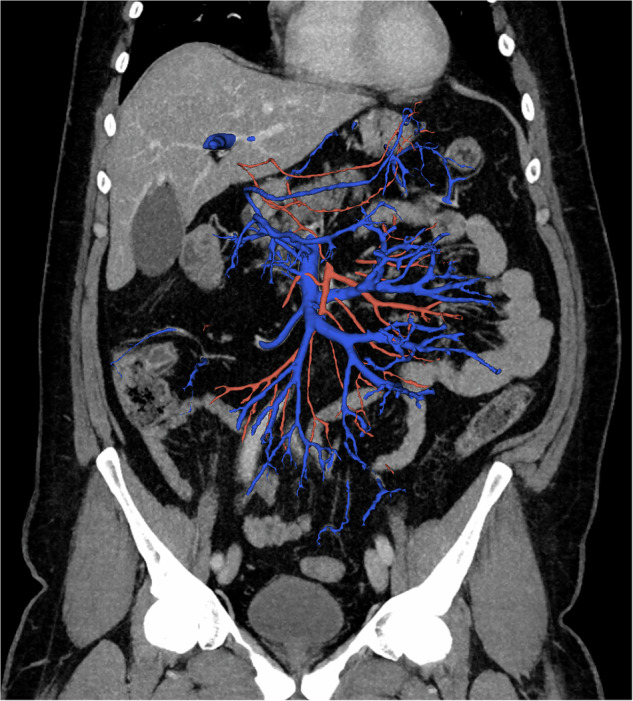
Manual mesenteric vessel annotations on dual-phase CT: arteries (red) from arterial phase and veins (blue) from venous phase, without inter-phase registration. Coronal and sagittal views highlight jejunal supply.
